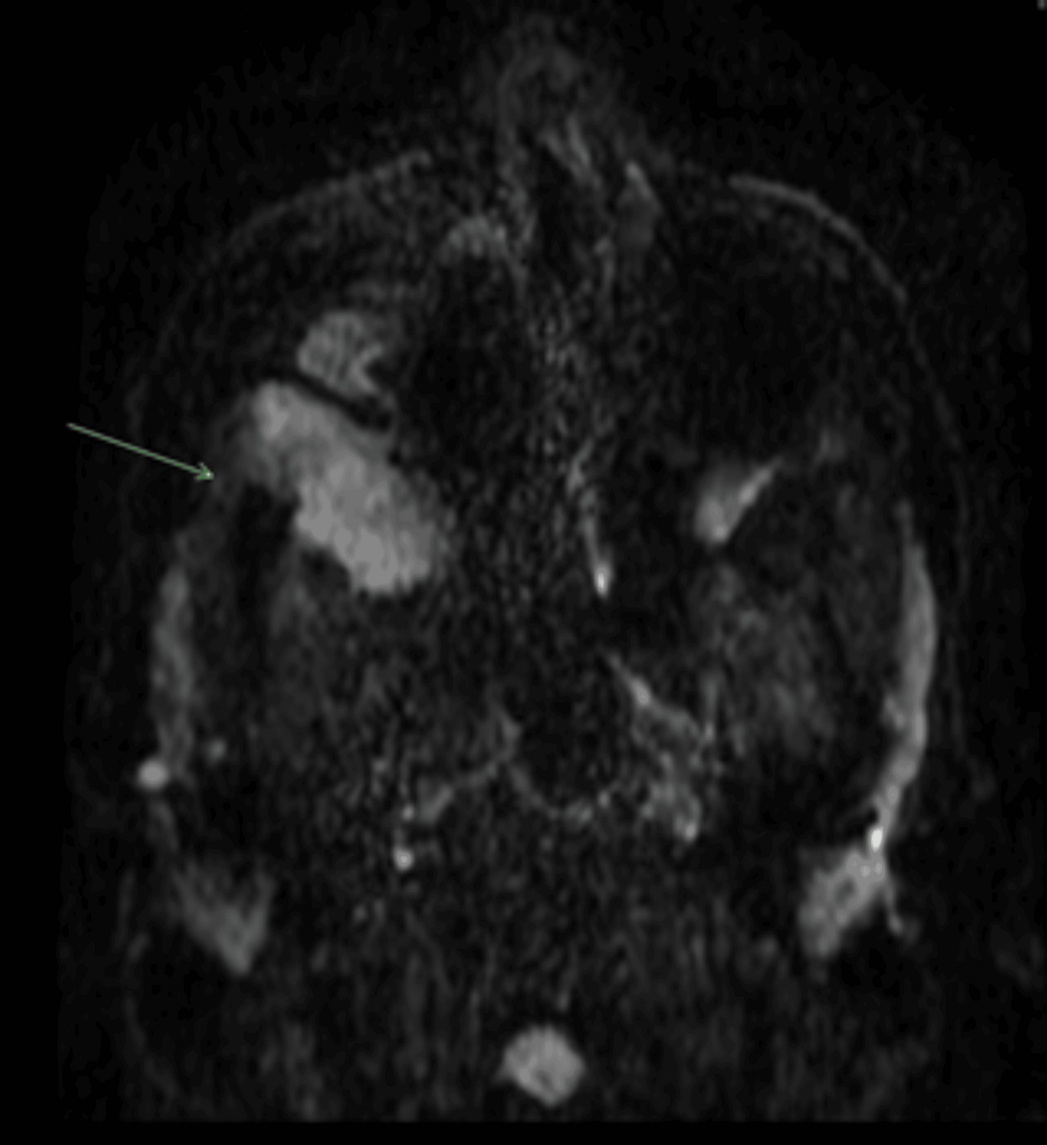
High b value diffusion weighted imaging (DWI) MRI Head and Sinus September 2022 Abnormal restricted diffusion within the tumour. Corresponding apparent diffusion co-efficient (ADC) map show low intralesional ADC values.
